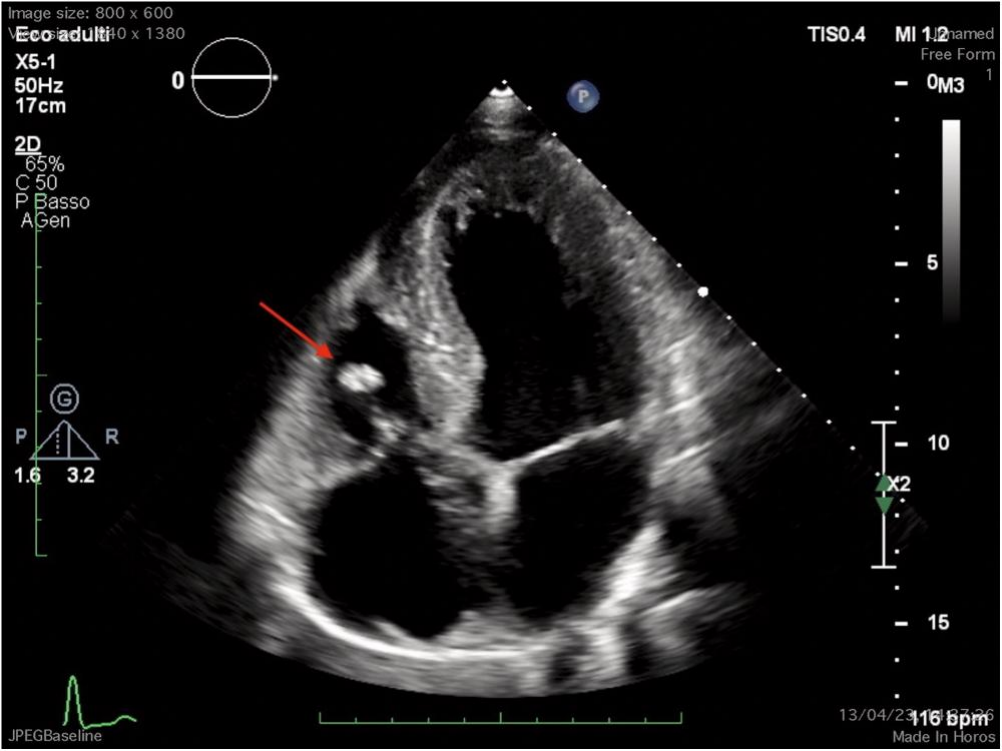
Transthoracic echocardiogram (apical four-chamber), vegetation on the tricuspid valve (arrow).
